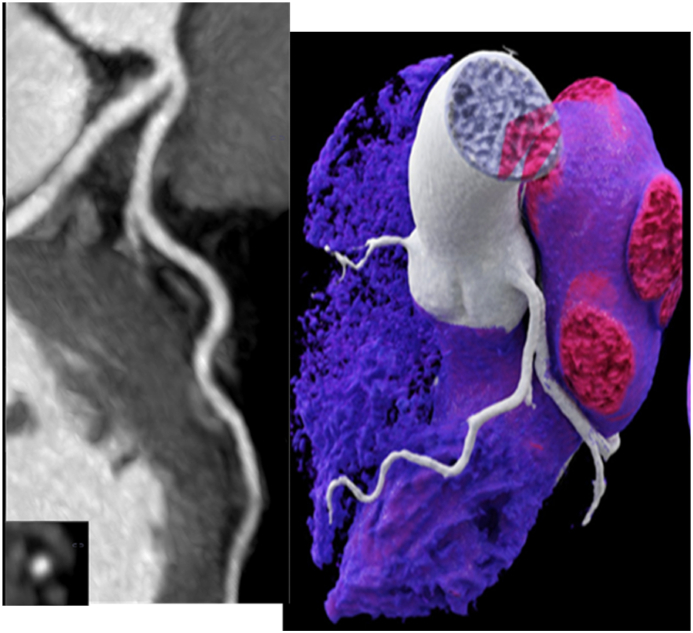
62-year-old female with hepatitis C, MELD Score 13, Child II stage, no alcohol since 30 years with post transfusion HCV after an accident, non-smoker since 34 years and no other risk factors. CTA ruled out coronary artery disease. LT was successful and outcome was excellent over 10 years. The 3DVRT (right) shows normal coronary arteries. CTA shows left anterior descending and circumflex artery (CX) (left, curved multiplanar reformation (cMPR)). CTA = computed tomography angiography; HCV = hepatitis C virus; LT = liver transplantation.
